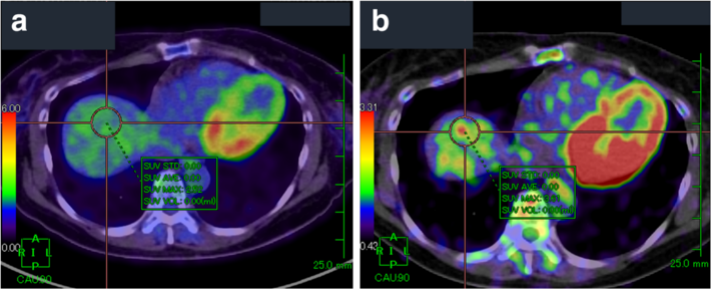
Axial slice on PET-CT showing the liver lesion in the early (a) and delayed phase (b). FDG uptake was undetectable among physiological uptake of the liver in the early phase; however, uptake elevated (SUVmax: 2.52 to 3.61) and was detectable in the delayed phase
